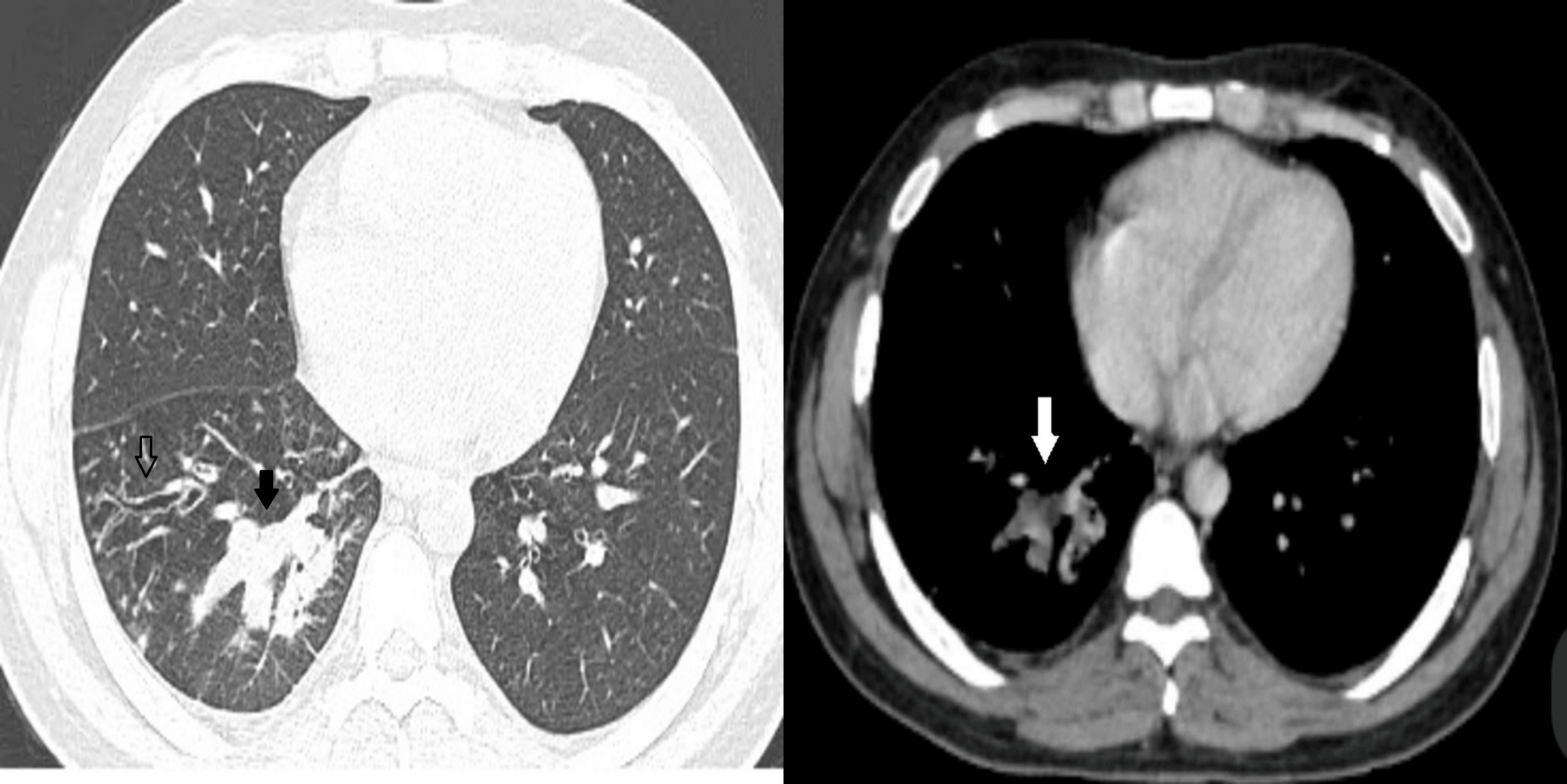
High-attenuation mucus (solid arrow) and bronchiectasis (open arrow) are seen in the axial image of the lung and mediastinal window of CECT of the thorax. CECT: contrast-enhanced computed tomography
